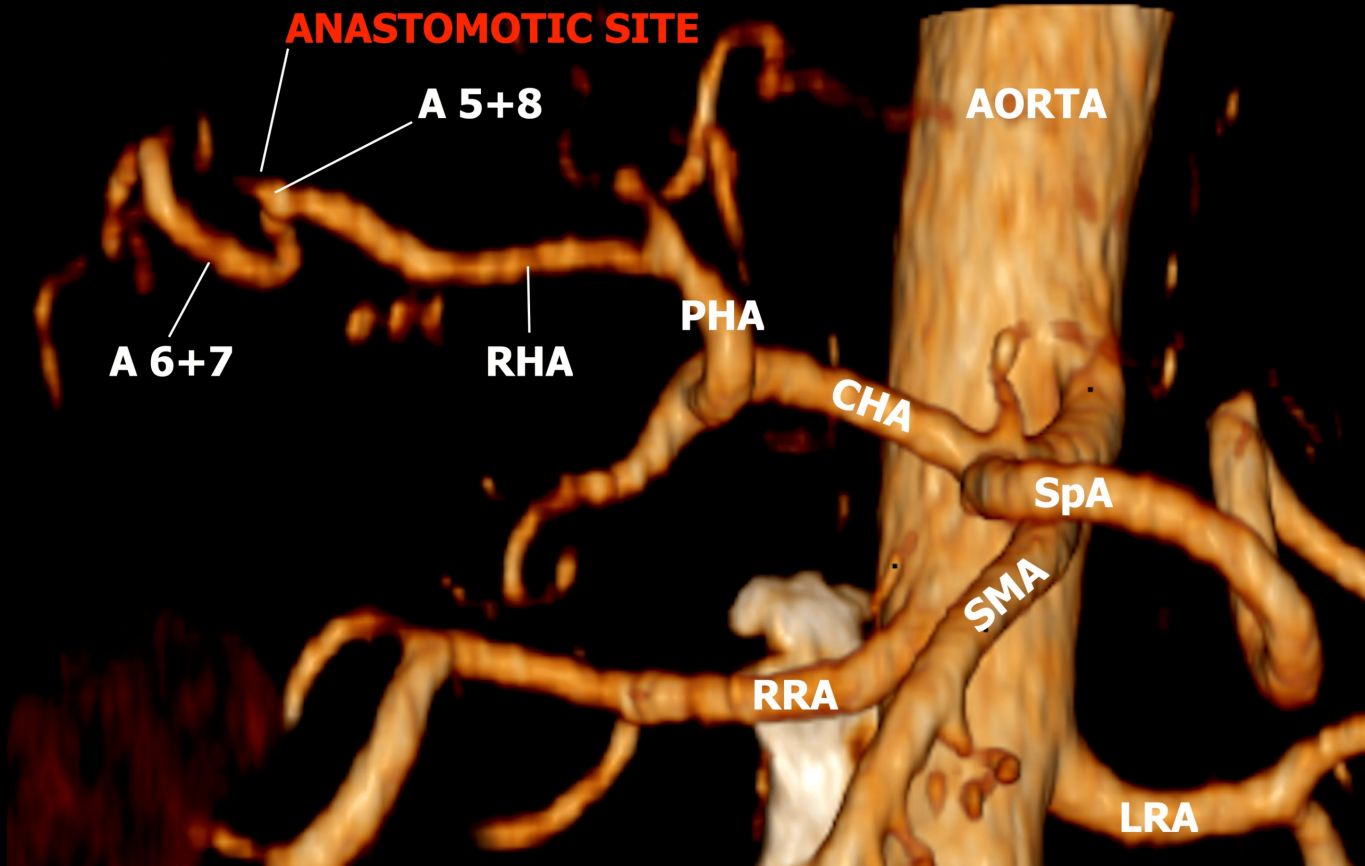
Absence of flow at the site of arterial reconstruction on CT angiography A 5+8: Anterior segmental artery of the liver; A 6+7: Posterior segmental artery; RHA: Right hepatic artery; PHA: Proper hepatic artery; CHA: Common hepatic artery; SpA: Splenic artery; RRA: Right renal artery; LRA: Left renal artery; SMA: Superior mesenteric artery.
